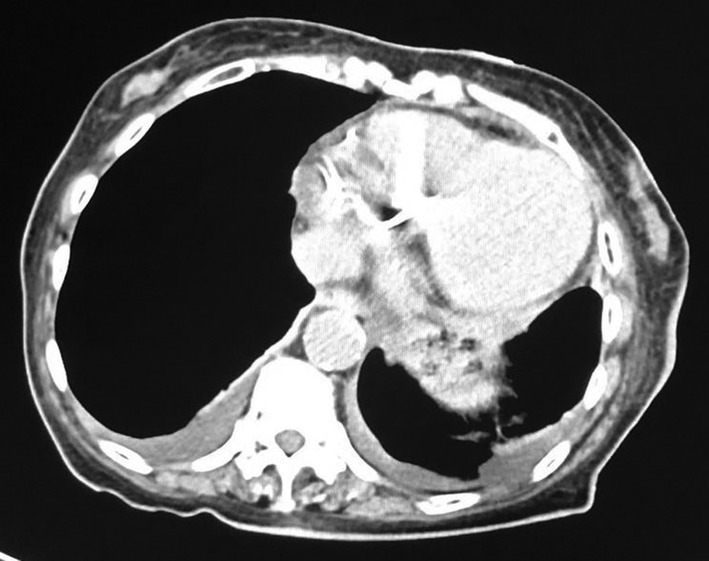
Computed tomography. Preoperatively, there was a small amount of pleural effusion.
